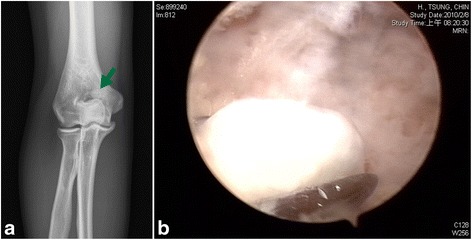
a Preoperative radiograph showing a loose body in the olecranon fossa (*arrow*). b Arthroscopic image showing removal of the loose body. c Arthroscopic image showing the olecranon fossa after debridement
